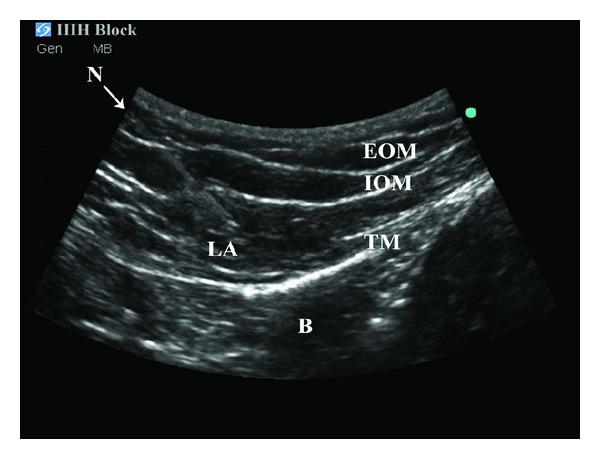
Sonographic anatomy of the US-guided IIIH block. N: needle, EOM: exterior oblique muscle, IOM: internal oblique muscle, TM: transversus muscle, LA: hypoechogenic local anesthetic pocket, B: bowel.
